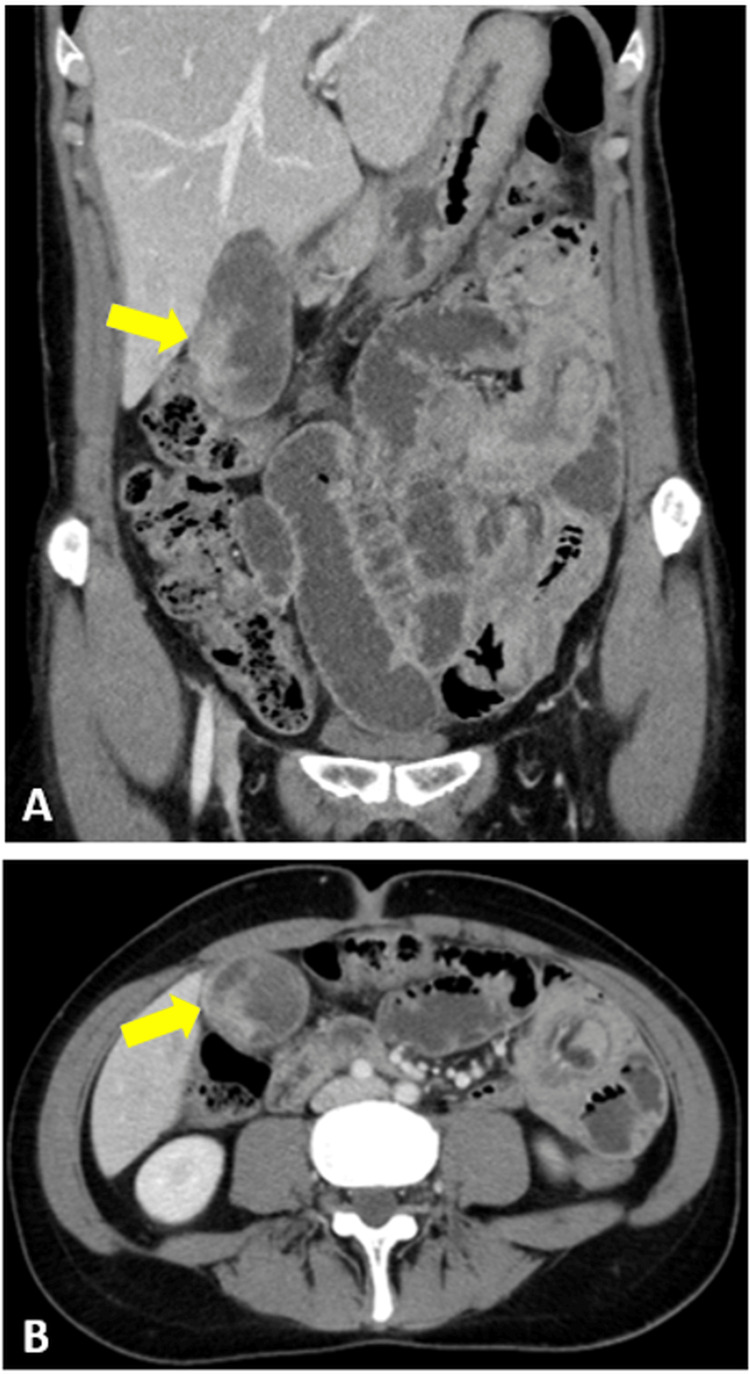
CT images on the day of Emergency Department admission demonstrating gallbladder haemorrhage (indicated by a yellow arrow). (A) Coronal plane; (B) Axial plane CT, computed tomography
